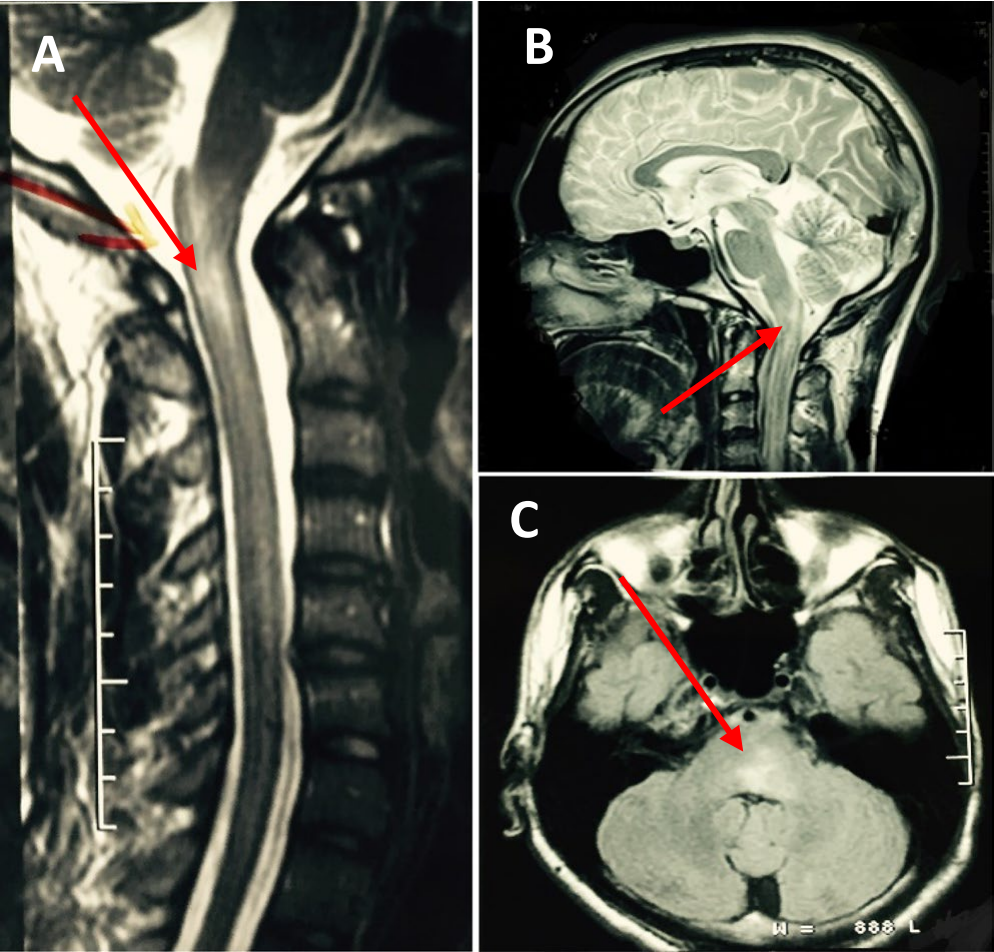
(A) Sagittal T2‐weighted MRI of the cervical spine: The image shows hyperintense lesions within the cervical spinal cord extending from the medulla to C5. (B) Sagittal T1‐weighted MRI: The image demonstrates a hyperintense lesion in the medulla and upper cervical region. (C) Axial T2‐weighted MRI: The image revealed a hyperintense lesion in pons.
